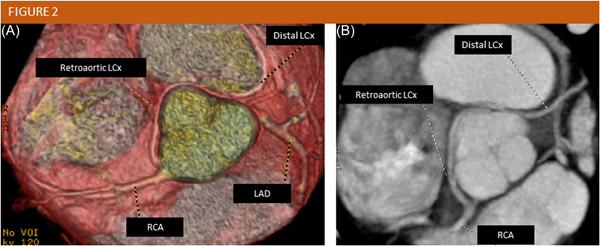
CCTA volume rendering (A) and maximum intensity projection (B) images showing a retro‐aortic left circumflex artery that arises from the right sinus of Valsalva. CCTA, coronary computed tomography angiography; LCx, left circumflex artery; RCA, right coronary artery.
